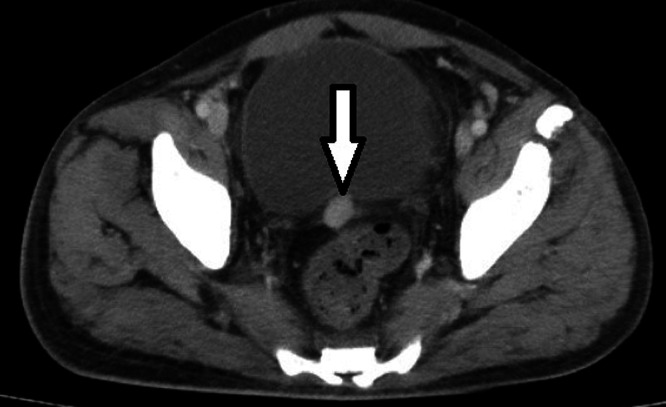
Axial contrast enhanced CT image showing splenic tissue implant in pelvis abutting posterior wall of urinary bladder.
